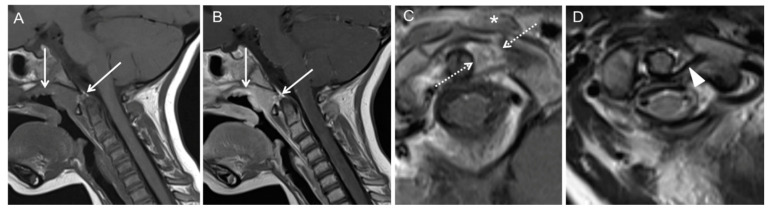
MRI shows edematous swelling and contrast enhancement of the posterior nasopharyngeal soft tissue with extension to anterior atlanto-occipital membrane and the cruciate ligament (A,B); arrows; 2D T1w TSE pre and post CA. Contrast enhancement of the left longus capitis (C); asterisk; 2D T1w TSE post CA signaling inflammation. No abscess formation present. Fixed rotation of C1 and C2 (C); dotted arrows; 2D T1w TSE post CA and synovial inflammation. Laxity of the transverse ligament of atlas (D); arrowhead; 2D T2w TSE. No spinal cord compression. T2w = T2-weighted; TSE = turbo spin echo; T1w = T1-weighted; CA = contrast agent.
